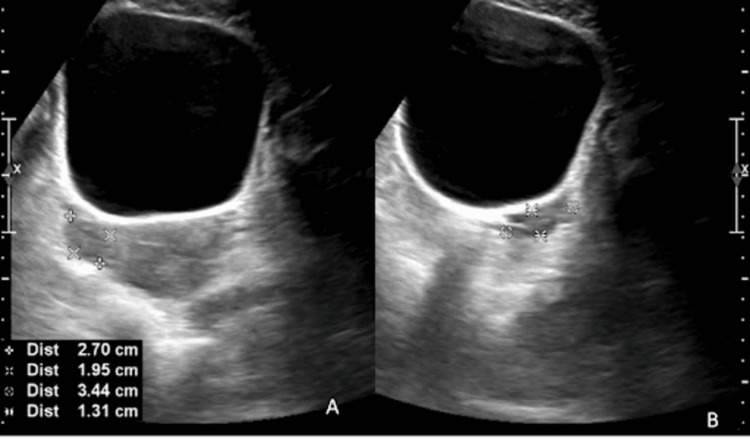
B-mode transabdominal ultrasound images reveal that the right (A) and left (B) ovaries are visualized separately.
